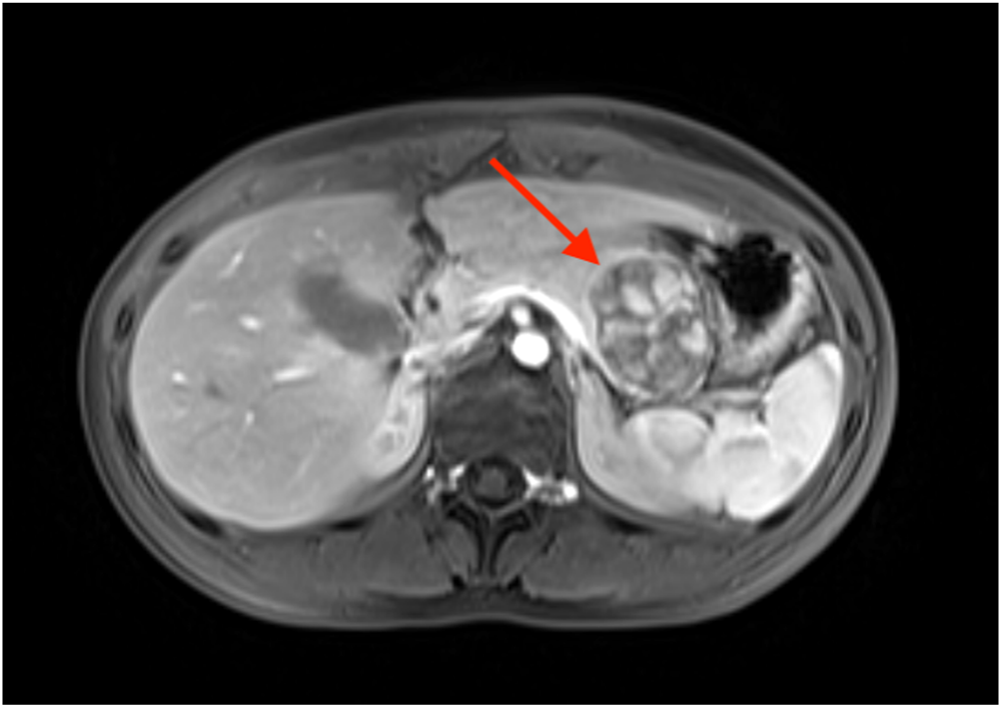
Axial fat‐saturated T1 post‐contrast magnetic resonance imaging (MRI) demonstrates a 4.5 × 3.8 cm well‐circumscribed mildly enhancing heterogeneous mass with internal papillary projections within the pancreatic tail.
